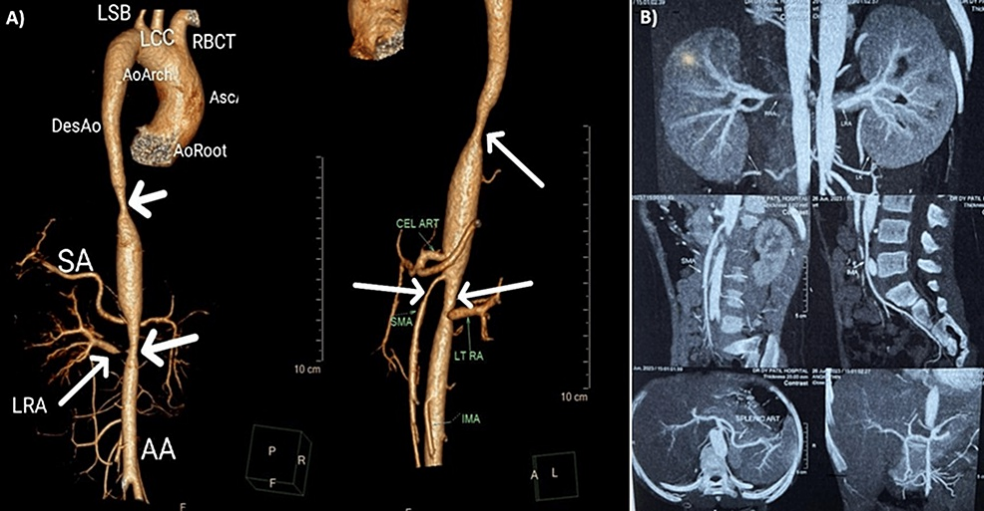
Radiology images confirming the diagnosis. CT aortogram showing (A) multiple segmental vascular stenosis with periaortic enhancing soft tissue and (B) wall thickening in the abdominal aorta, lower thoracic aorta, origin of bilateral renal arteries, right subclavian artery, and right axillary artery. LSB: left subclavian artery; LCC: left common carotid artery; RBCT: right brachiocephalic trunk (also known as innominate artery); DesAo: descending aorta; ASC: ascending aorta; AoRoot: aortic root; SA: subclavian artery; LRA: left renal artery; AA: abdominal aorta; CEL ART: celiac artery; SAM: superior mesenteric artery; IMA: inferior mesenteric artery
